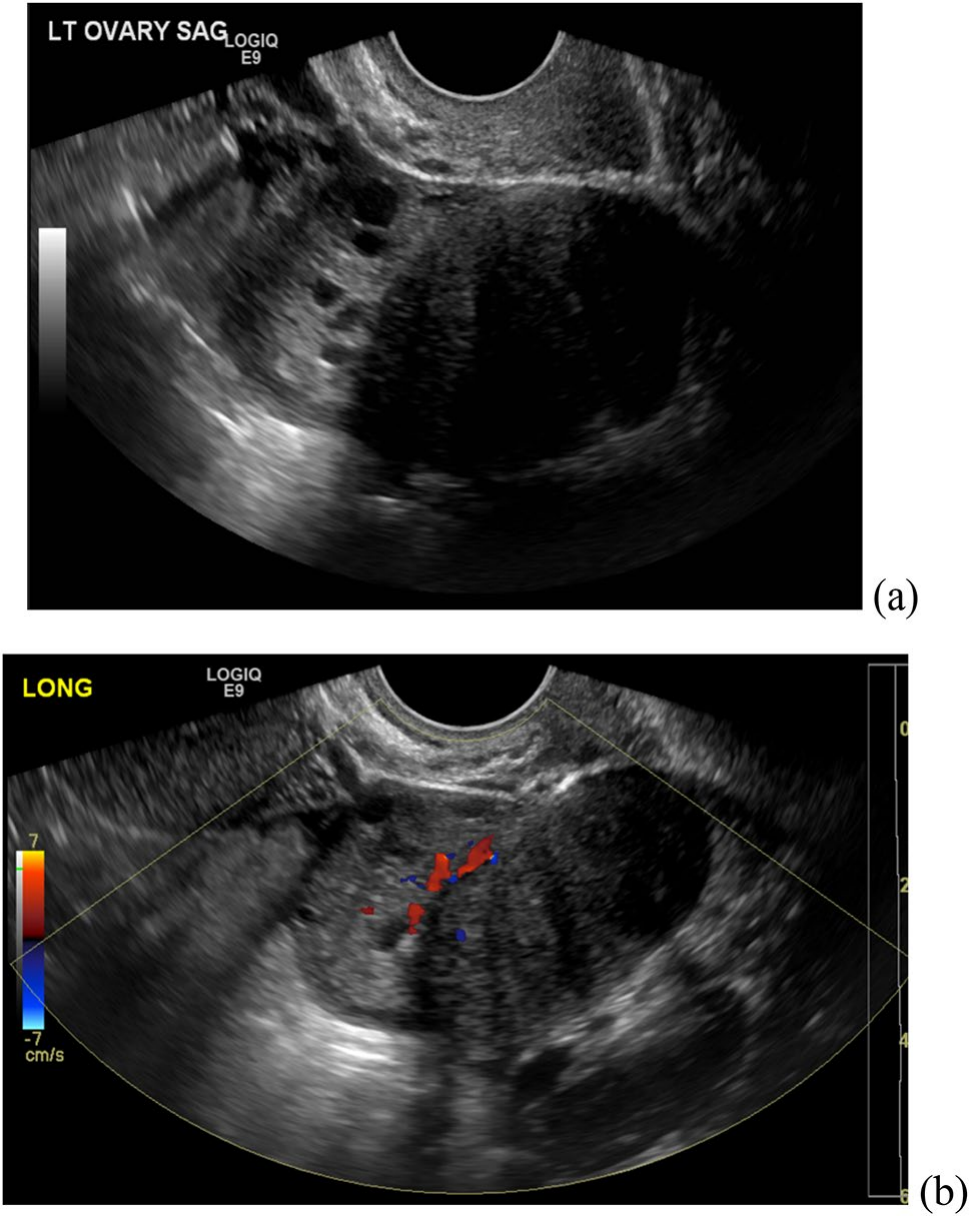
A 21-year-old woman with no significant past medical history, who presented with menstrual irregularity and pelvic pain. Pelvic ultrasound demonstrated a solid left ovarian mass (image a) with internal vascular flow (image b), most consistent with a solid ovarian lesion such as fibrothecoma
